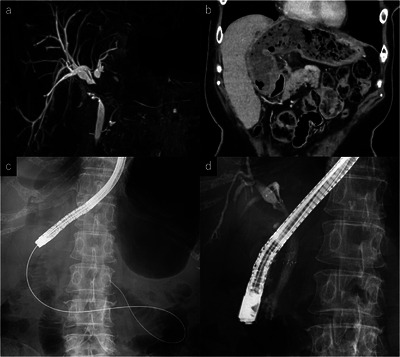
(a) MRCP showing hilar biliary stricture (Bismuth II) due to peritoneal dissemination, with dilatation of the intrahepatic bile ducts. MRCP: magnetic resonance cholangiopancreatography. (b) CECT demonstrated gastric deformation, with the distal stomach adherent to the remnant liver. CECT: contrast‐enhanced computed tomography. (c) Because of gastric deformation, a standard duodenoscope could not be advanced into the second portion of the duodenum. A forward‐viewing scope was used to insert a guidewire into the distal duodenum, and the duodenoscope was advanced over the wire. (d) Side‐by‐side placement of MHSEMSs into the right anterior and posterior segmental bile ducts for hilar biliary stricture. MHSEMSs: multi‐hole self‐expandable metal stents.
